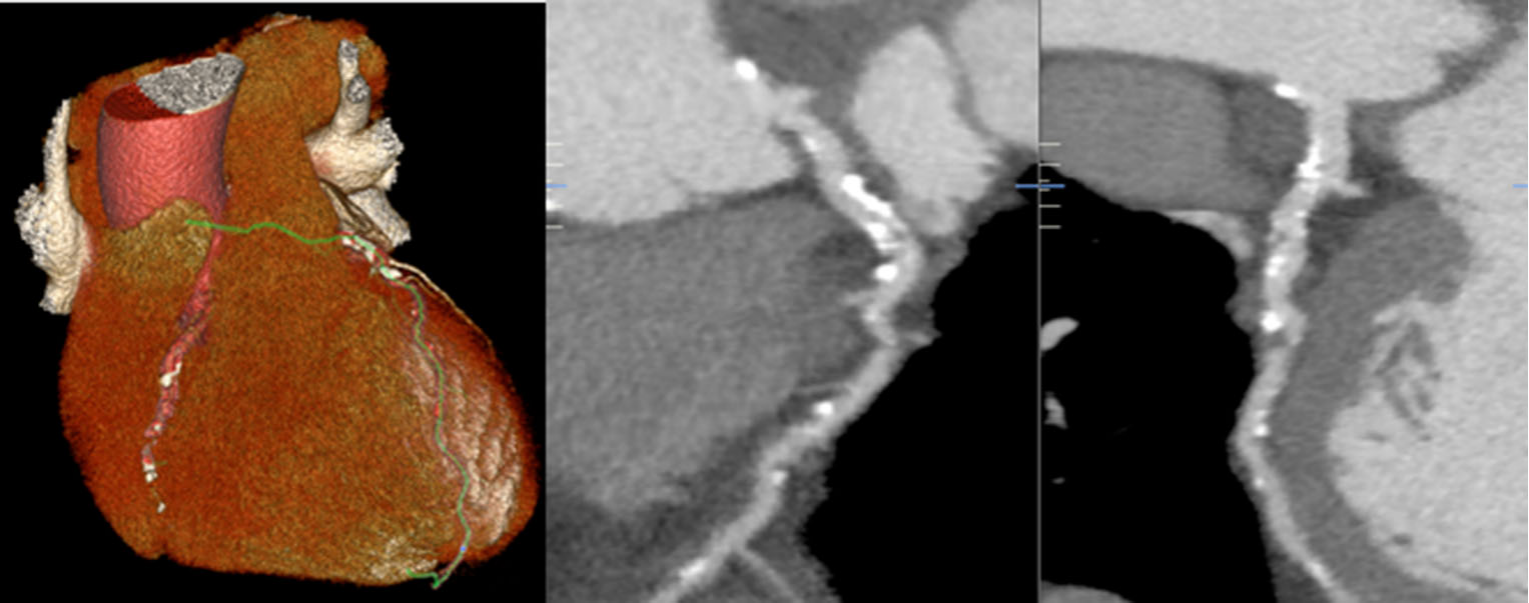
Example of coronary computed tomography angiography (CCTA) image in a 55-year-old gentleman with a calcium Agatston score of 1400
